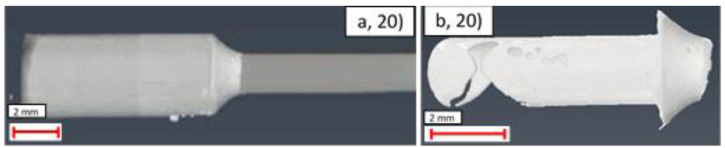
CT images of Ni-coated sample (20): (a) side view of pipe and gland exterior; (b) side view of left side interior solder.
